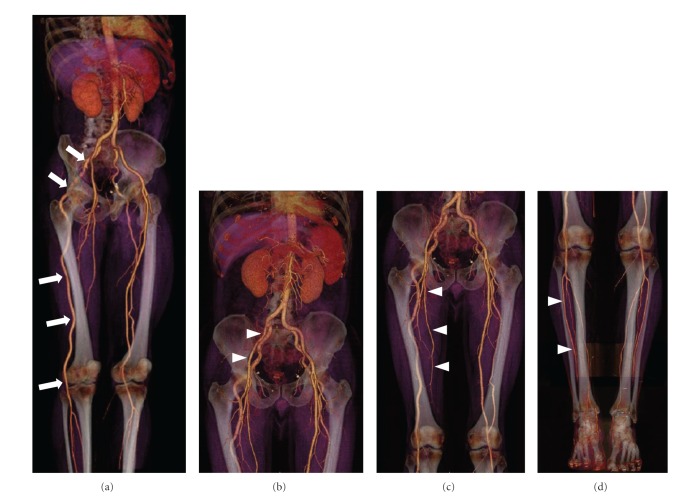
(a) Volume Rendering reconstruction of CT-A study. On the right side a large abnormal artery originates from a hypertrophic internal iliac artery and run along the gluteal region and the posterior thigh supplying the entire vascular axis of the leg (arrows). ((b)-(c)) The external iliac, the deep femoral and the superficial femoral arteries showed a slightly reduced caliber but no obstruction (arrowheads). (d) The circulation of calf and foot are regularly patent (arrowheads).
